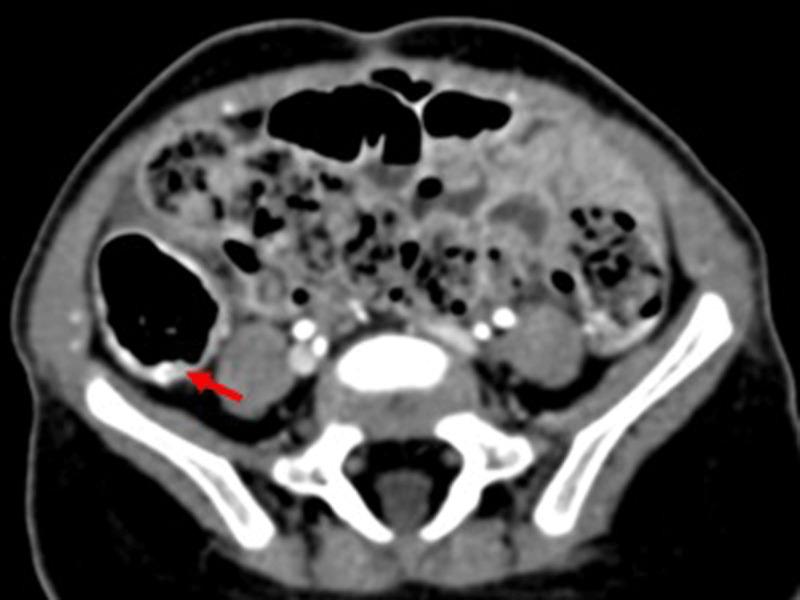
Abdominal CT image of the abdomen (arterial phase) 12 months after treatment with propranolol showed almost complete regression of the bowel wall thickening, with slight arterial phase hyperenhancement on the cecal wall as the only finding.
